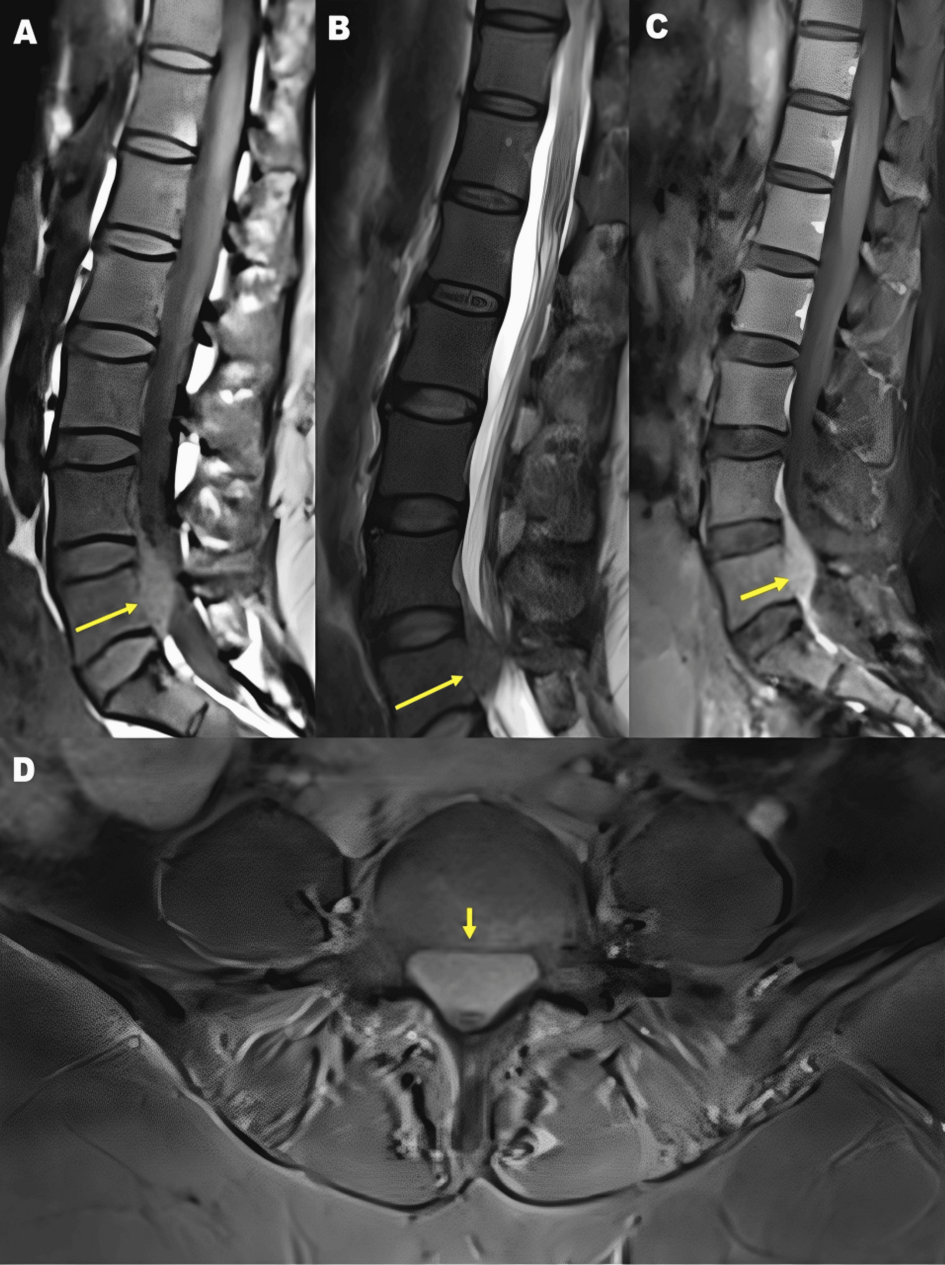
(A) Sagittal T1-weighted MRI of the lumbosacral spine at L5 showing an isointense anterior epidural lesion causing posterior displacement of the thecal sac. (B) Sagittal T2-weighted MRI at L5 demonstrating the lesion with mild hyperintensity and persistent thecal sac compression. (C) Sagittal post-contrast T1-weighted MRI showing avid enhancement of the anterior epidural lesion. (D) Axial post-contrast T1-weighted MRI at L5 demonstrating a homogeneously enhancing anterior extradural mass with thecal sac compression.
